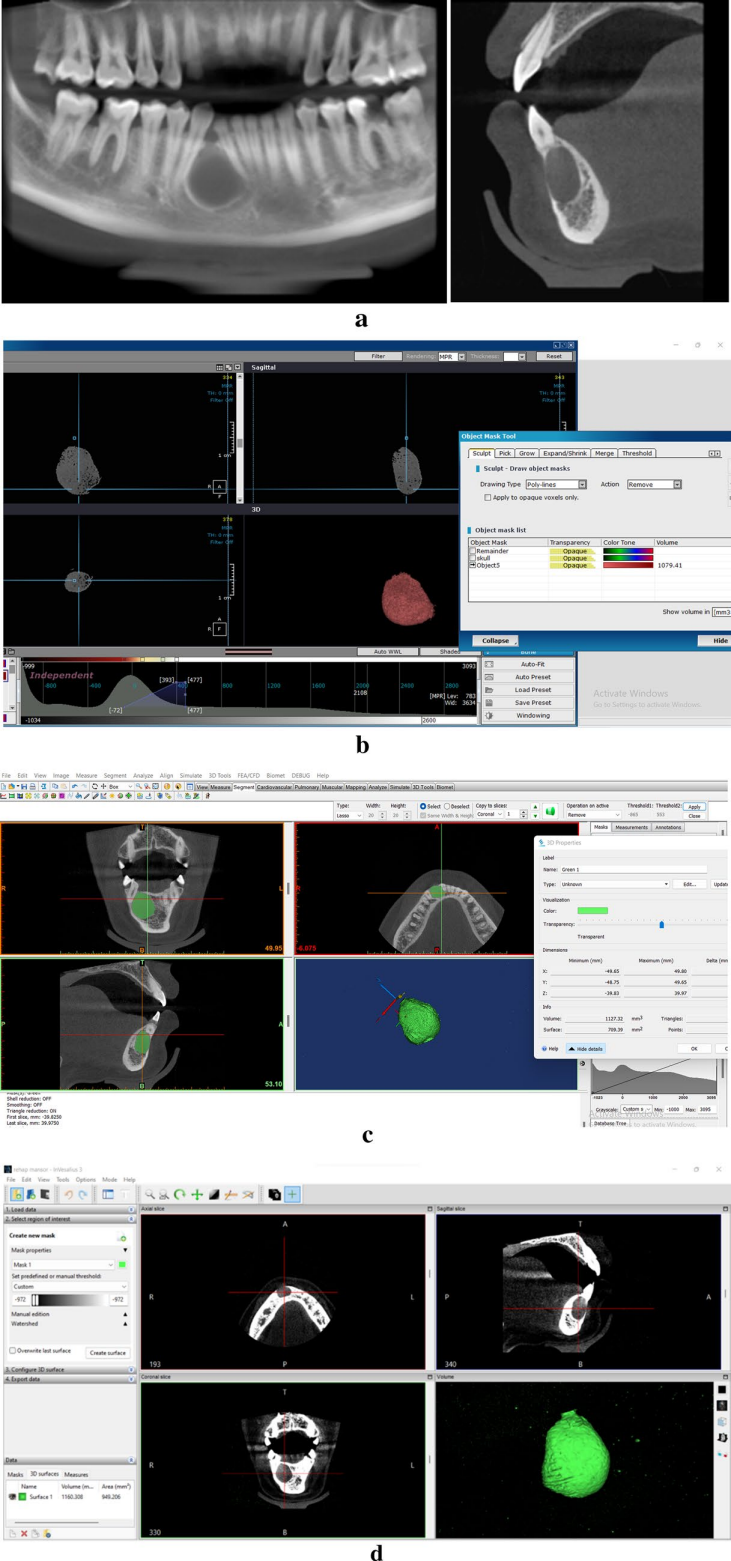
CBCT reformatted panoramic view (1a) and sagittal cut of a lateral periodontal cyst (2a), OnDemand volume analysis of the cystic lesion (b), MIMICS volume analysis of the cystic lesion (c), InVesalius volume analysis of the cystic lesion (d)
